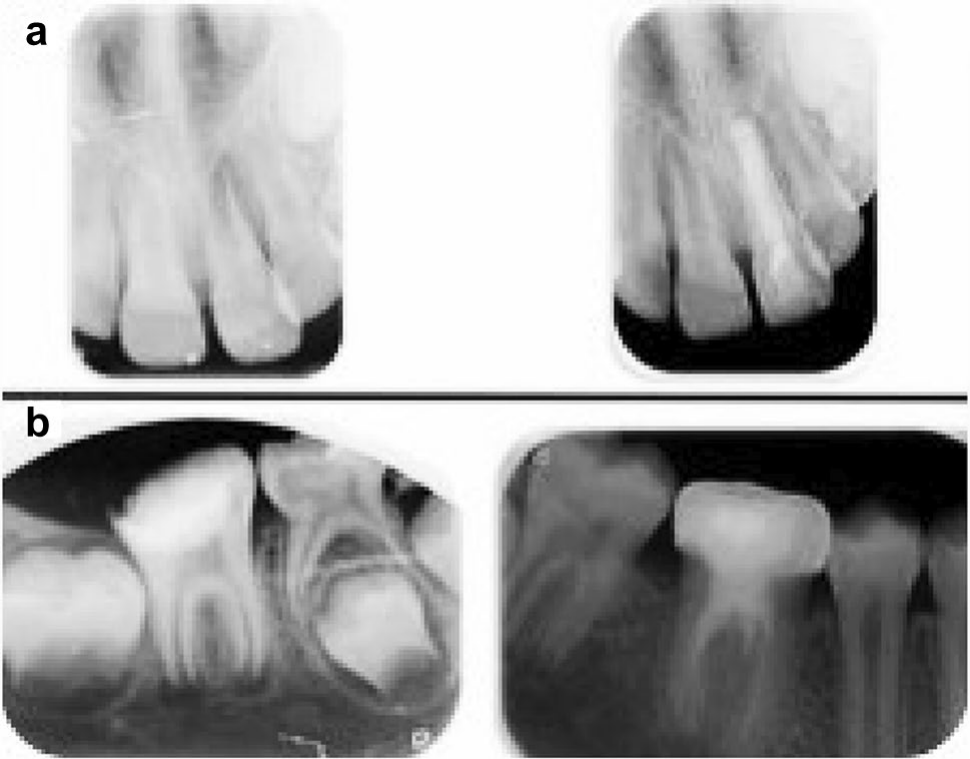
Radiographic apical healing success with MTA apical plugging (a) and regenerative endodontic treatment (b)
